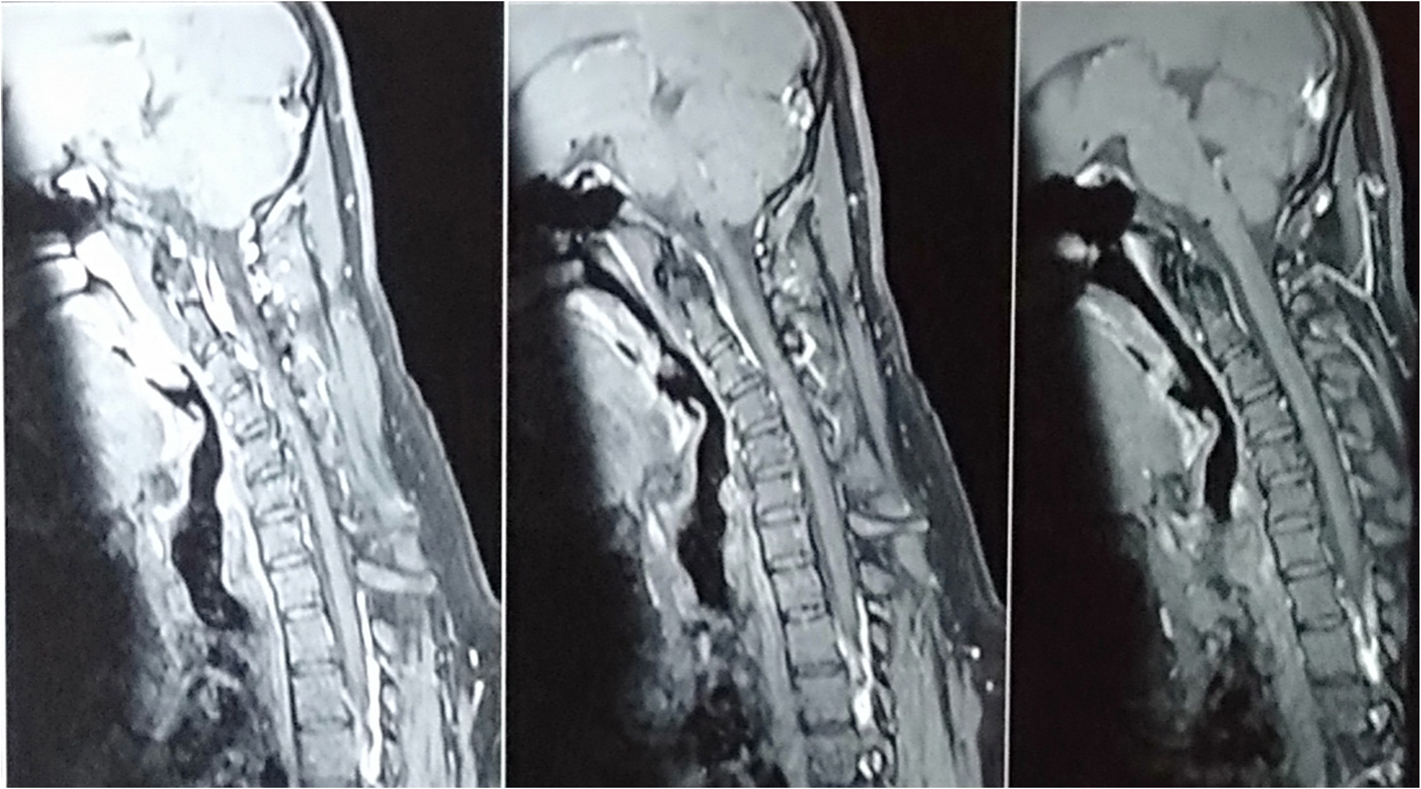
Sagittal T1-weighted contrast with cervical flexion images without epidural enhancement or cord displacement
